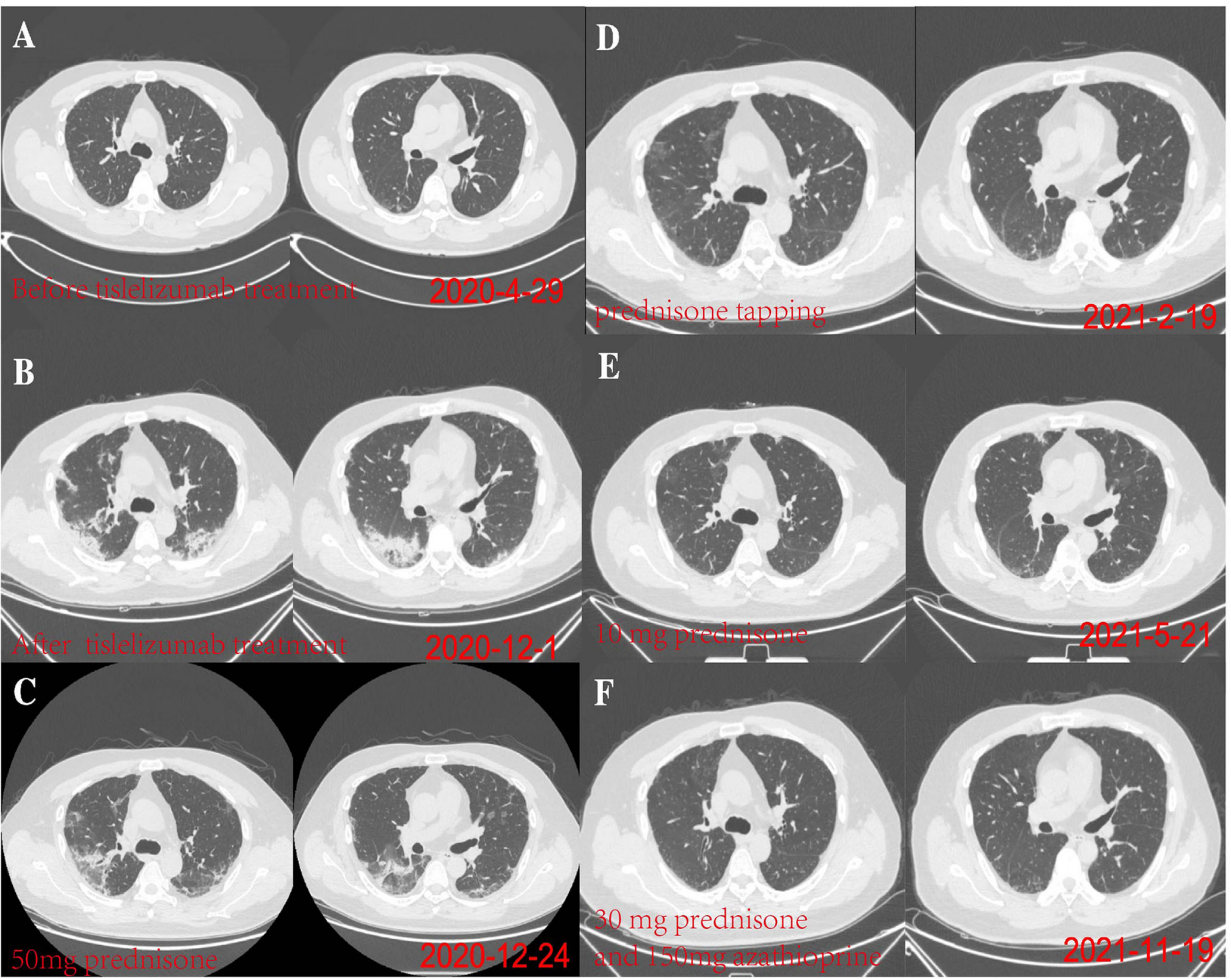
(A) On April 29, 2020, chest computed tomography (CT) before chemotherapy showed mild ground glass opacity in the lower lobe of the right lung. (B) On December 1, 2020, after 6 months of tislelizumab treatment, chest CT showed diffuse ground glass and solid opacity in both lungs, more severe in both lower lungs. (C) On December 24, 2020, after 3 weeks of prednisone treatment, chest CT showed pulmonary infiltration had improved. (D) pulmonary infiltration continued to improve on Feb 19, 2021. (E) Multiple subpleural ground glass lesions recurred in both lungs on May 2, 2021 (The patient received 10 mg prednisone). (F) The patient responded to azathioprine (150 mg per day) on November 19, 2021.
